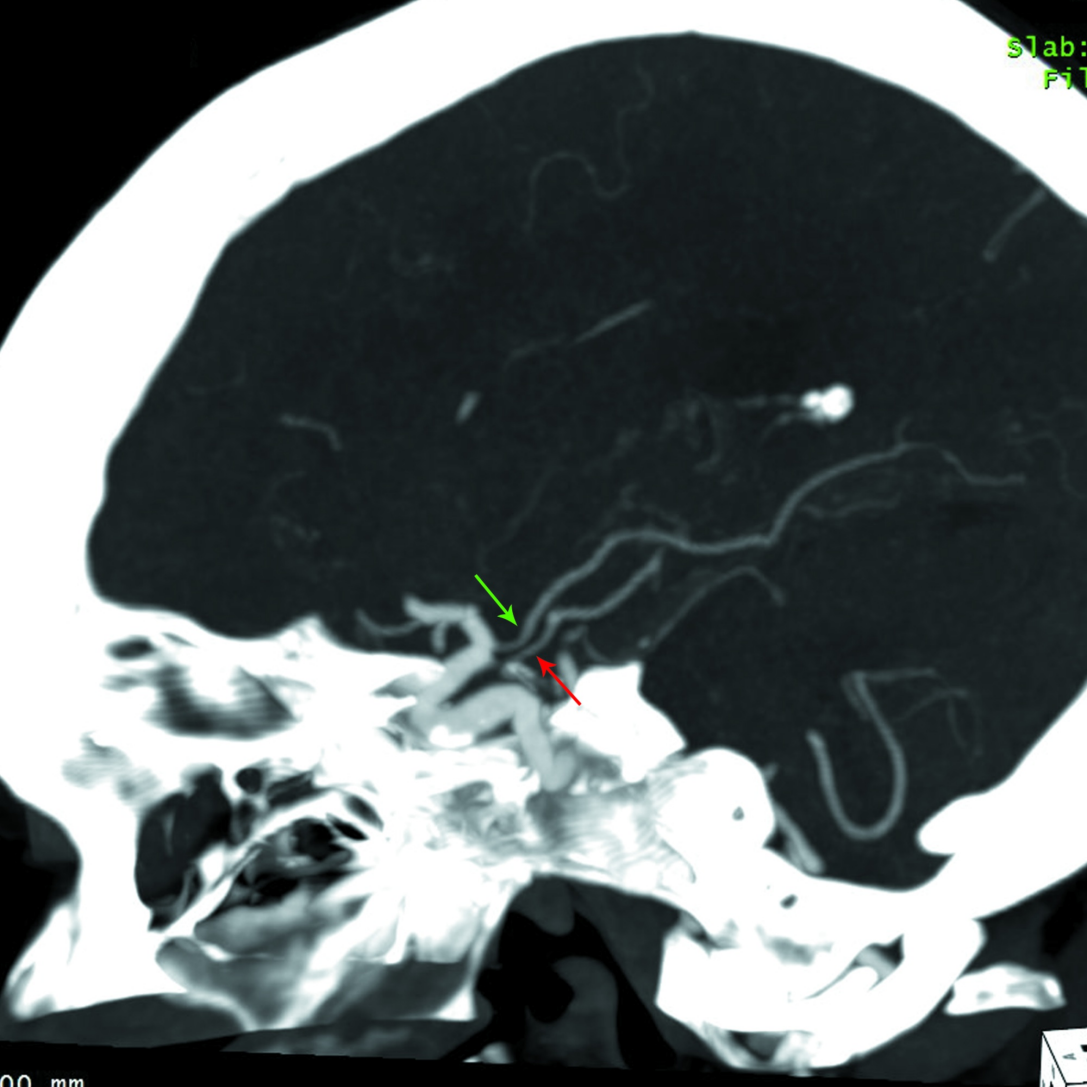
Sagittal MIP reformatted images from CT angiogram of the head showing the course of the right ICA A fetal PCA arises from the posterior aspect of the ICA and has a normal posterior course (green arrow). An additional vessel arising in close proximity but proximal to the fetal right PCA parallels its course until the P2/3 segment, where it ascends, crossing the PCA, and continues to terminate within the choroid plexus in the right lateral ventricle (red arrow). MIP = maximal intensity projection; CT = computed tomography; ICA = internal carotid artery; PCA = posterior cerebral artery
